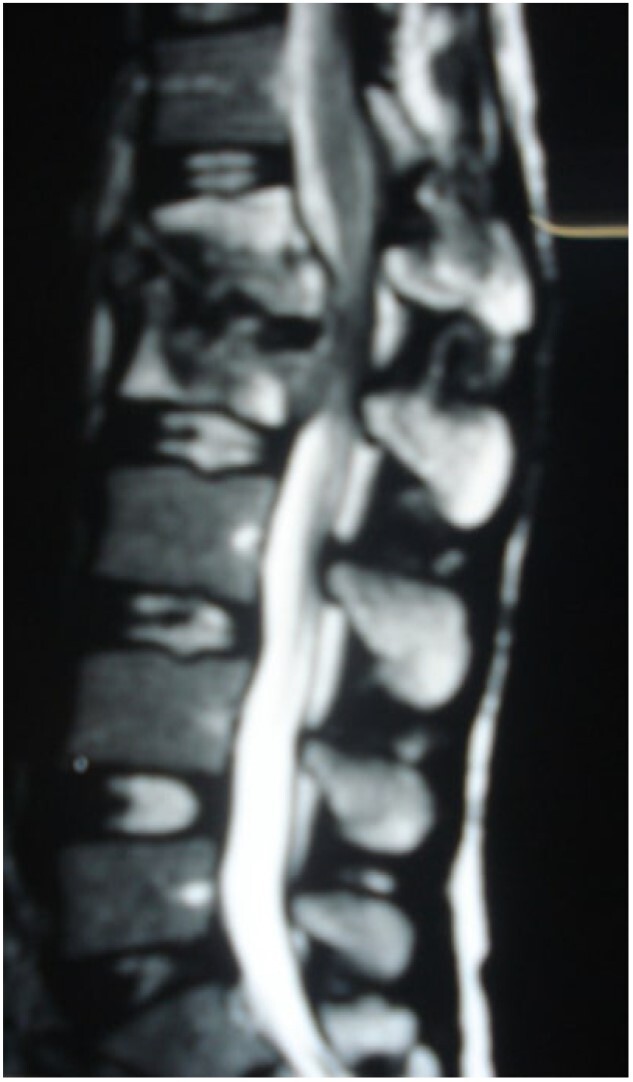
Sagittal T2-weighted MRI shows severe disco vertebral destruction, the formation of epidural abscess and compression of the spinal cord at the L1–2 level.
